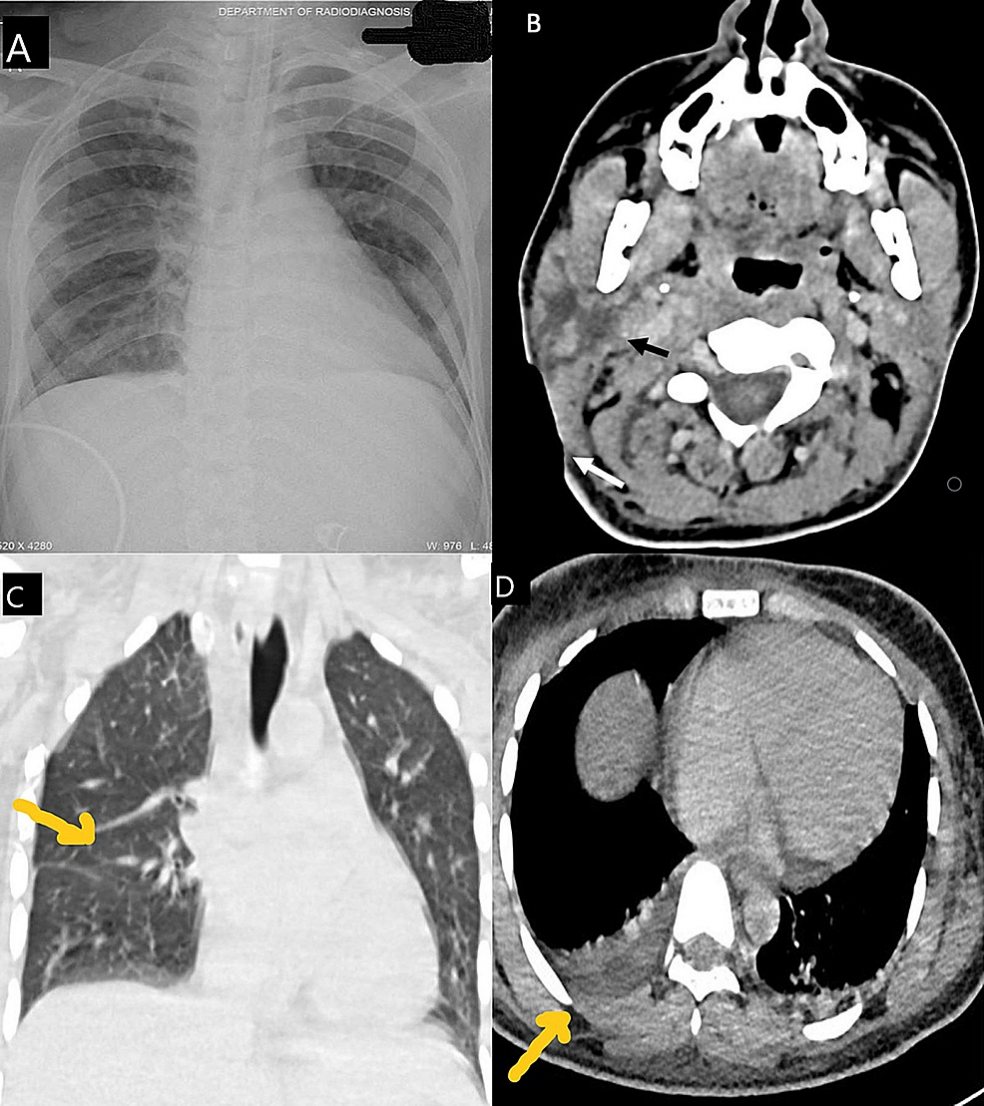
Radiology findings. A: X-ray of the chest: grossly normal. B: CECT of the neck (axial): an arrow showing the irregular, well-defined, non-enhancing area into the neck spaces extending to the parotid area, and another arrow showing the soft-tissue defect in the neck region. C: CECT of the chest (coronal): lung window image revealing normal bilateral lung parenchyma. D: CECT of the chest (axial): mediastinal window image showing a mild right-sided pleural effusion. CECT: contrast-enhanced computed tomography
